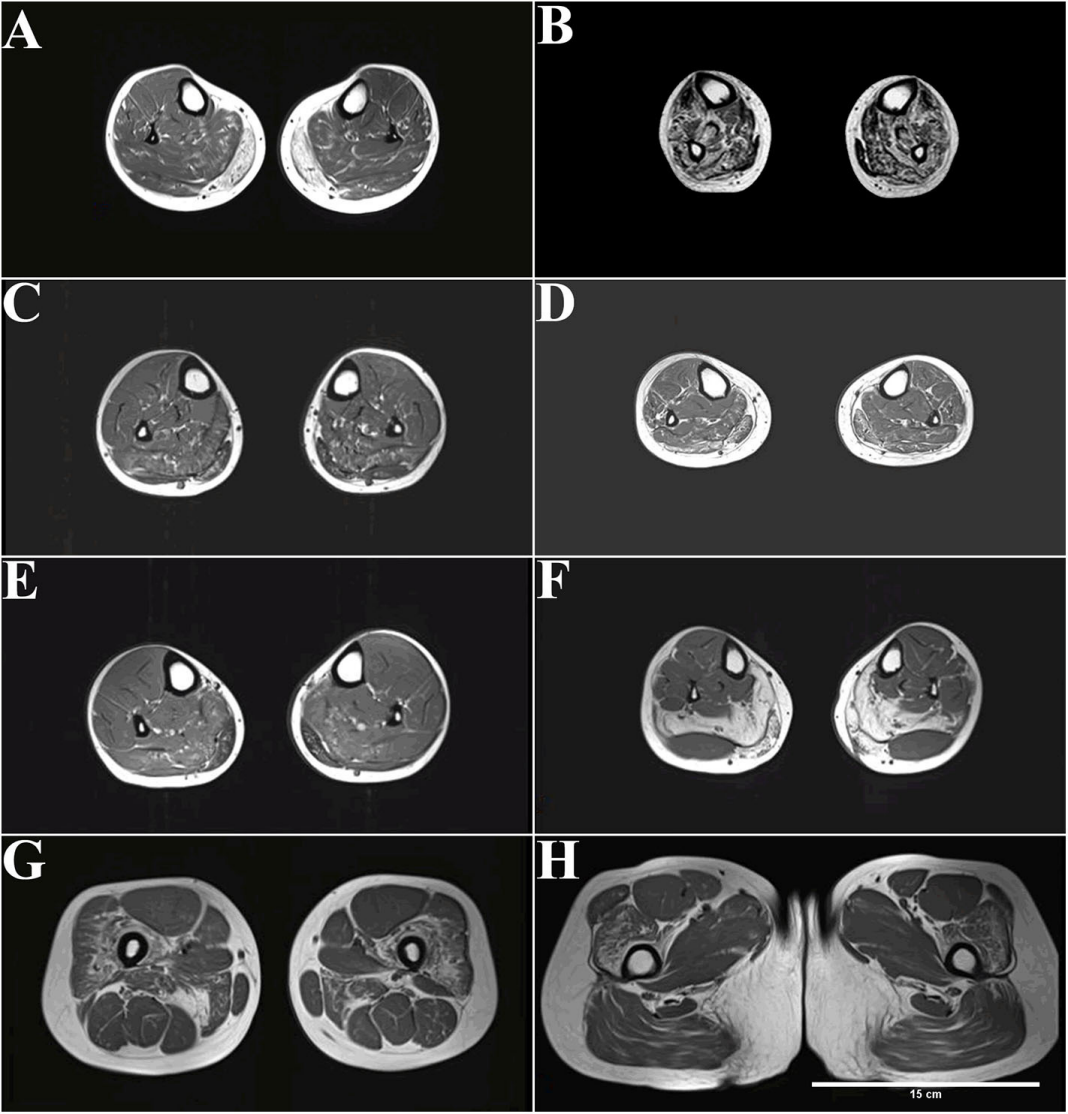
MRI (T1-weighted) of patients with dysferlinopathy shows fatty infiltration of muscle. (A) The peroneus, extensor digitorum longus, and extensor halluces longus muscles were involved in patient 1 with disease duration of 4 years. (B) More advanced stages show an involvement of the tibialis anterior in patient 2 with disease duration of 20 years. (C,D) The peroneus, extensor digitorum longus, and extensor halluces longus muscles were also involved in patient 3 (C) and patient 4 (D) with disease duration of 3 years. (E,F) Only the gastrocnemius and soleus muscles were affected in patient 6 (E) and patient 7 (F) with disease duration of 1–2 years. (G,H) Increased signal can be observed in the quadriceps femoris and adductor magnus muscle in patient 7.
